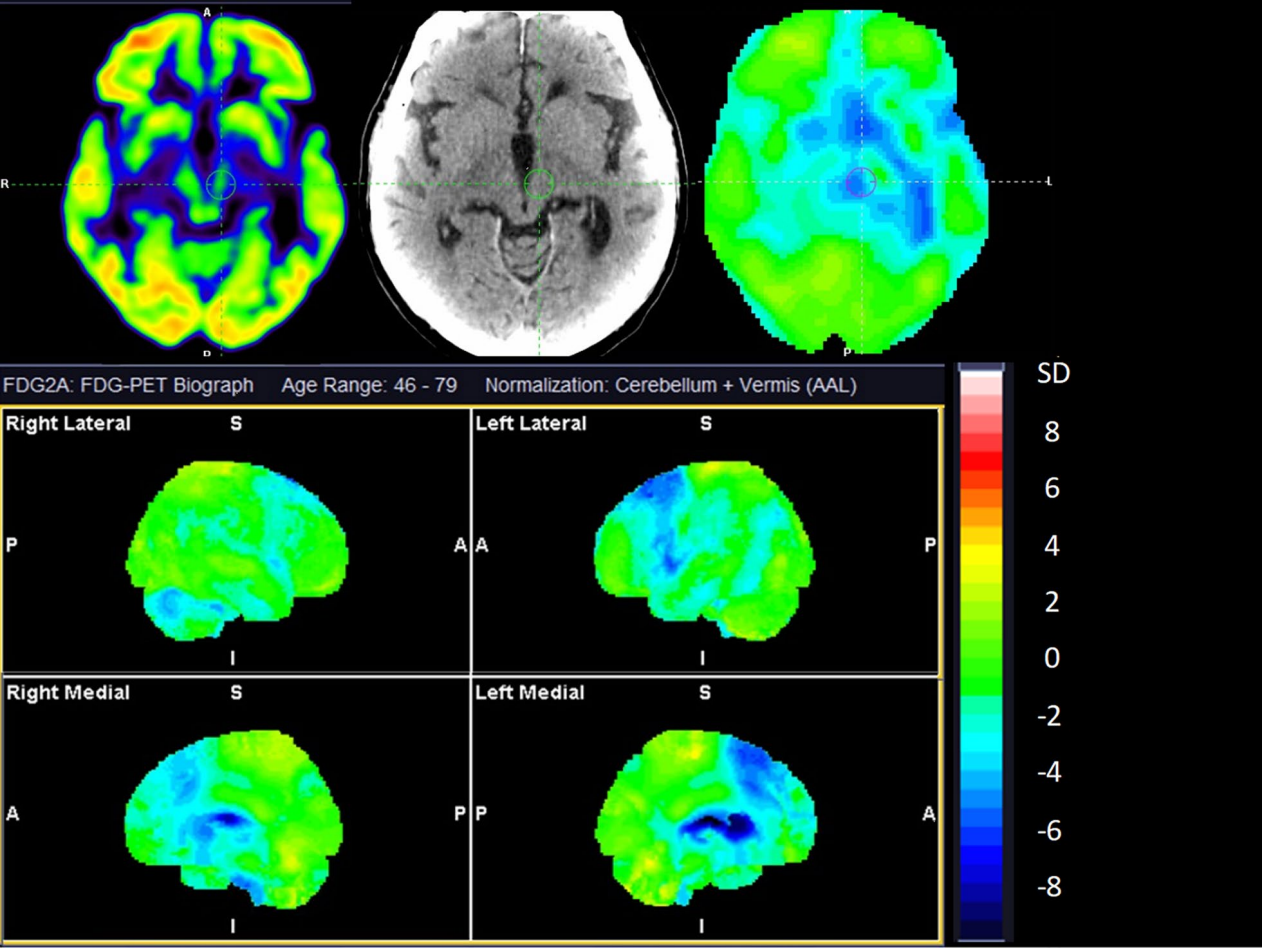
Cerebral [^18^F]FDG PET of a 73-year-old man with overlapping features of CBD and PSP. Top row show axial sections at the level of the basal ganglia and mesencephalon of [^18^F]FDG, CT, and statistical maps. The bottom rows show statistical surface projections with standard deviations from healthy subjects. Please note the asymmetry and involvement of the basal ganglia pointing towards CBD and the involvement of mesencephalon and mesial frontal cortex pointing towards PSP
